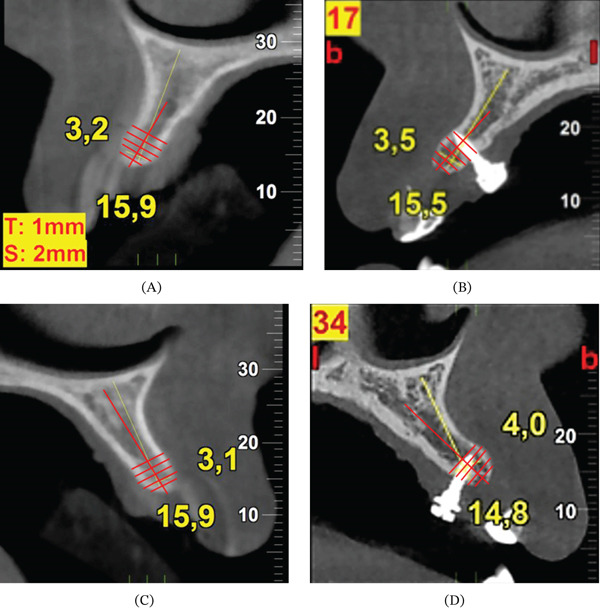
(A–D) Comparative CBCT images (measured).
